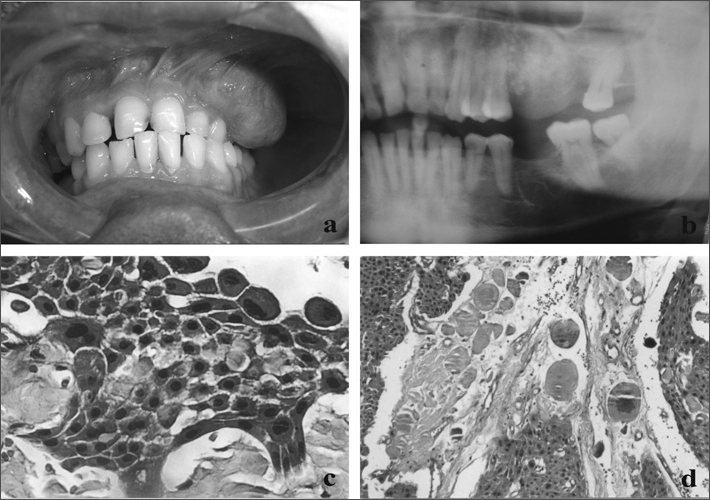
(a) Exophytic nodular lesion in the maxillary vestibular gingiva; (b) panoramic radiograph showing a radiolucent lesion of non-distinct borders and central radiopacities; (c) epithelial polyhedral cell islands with pleomorphism and prominent intercellular bridges (HE/ 400x); (d) concentric lamellar calcifications among epithelial polyhedral cell islands (HE/ 100x).
